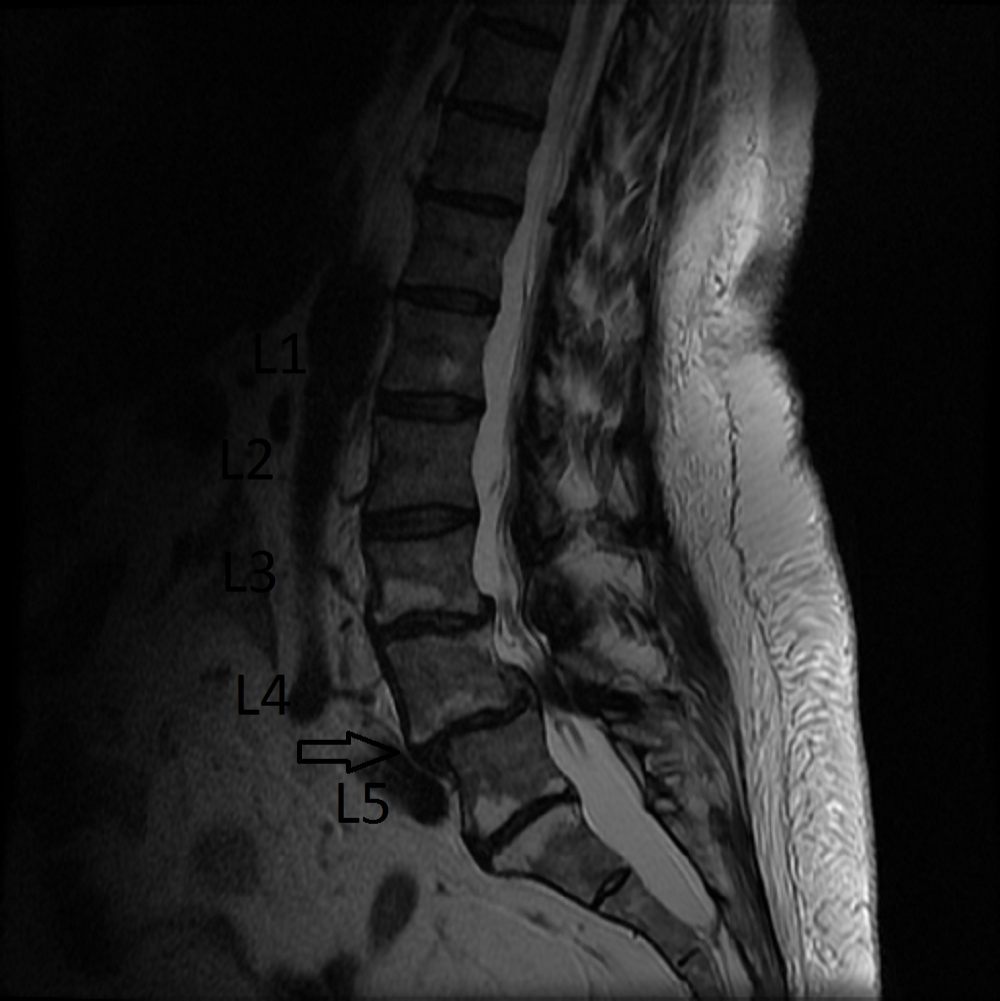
Pre-op Midsagittal Section: MRI of Lumbar Spine
